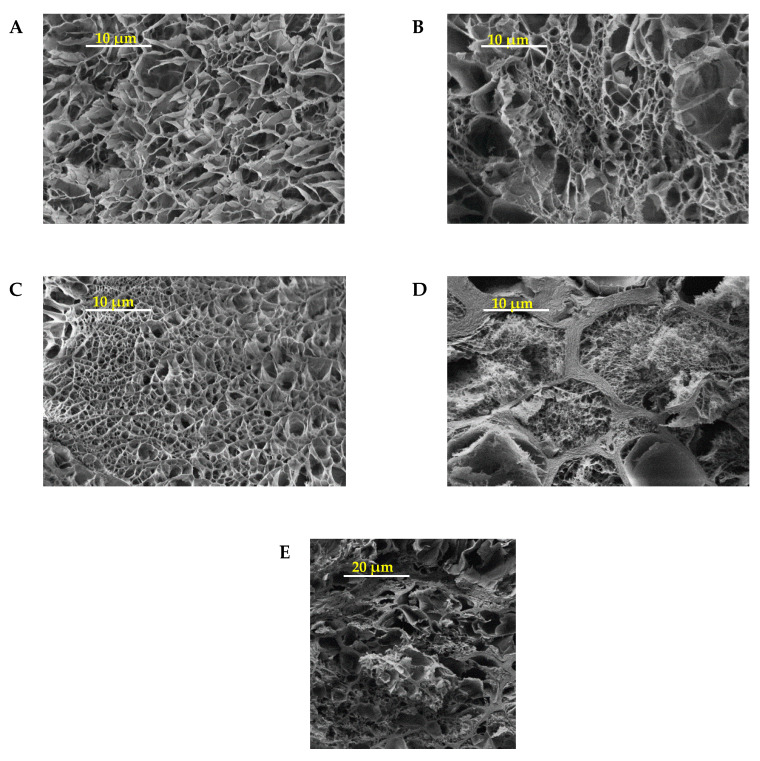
SEM images with 2000-fold magnification of (A) NC-N, (B) NC-NT, (C) NC-C and (D) NC-CT gels. Scaling bar represents 10 µm. (E) 500-fold magnification of NC-CT gels. Scaling bar represents 20 µm.
